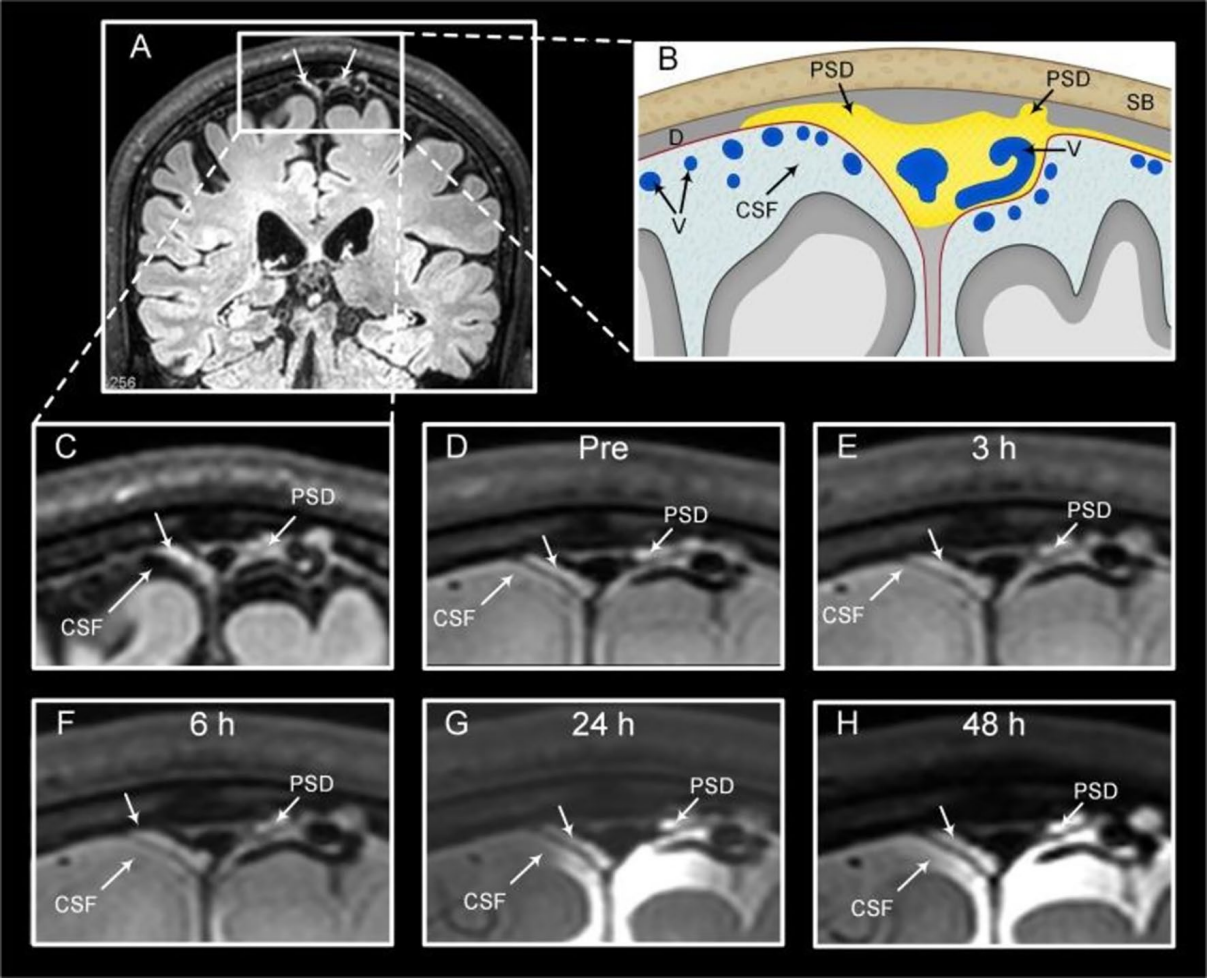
The parasagittal dura (PSD) is visible as hyperintense tissue at FLAIR (A and C). PSD (B) volume varies widely between subjects for unknown reasons, but has in addition to stroma been shown to contain arachnoid granulations, blood vessels, intradural channels, lateral lacunae and lymphatic vessels, the latter at micrometer scale. After injection of a CSF tracer (MRI contrast agent), PSD enhances visibly with tracer after 24 and 48 h in a diffuse fashion, but not in the shape of tubular structures. Tracer enhancement in PSD is dependent on tracer enhancement in the adjacent cerebrospinal fluid (CSF) space and is not visible before tracer has reached the CSF compartment (D–F). At 24 h (G) and 48 h (H),  From Eide PK and Ringstad (Brain Research) [28], reused with permission under the terms of the Creative Commons CC-BY license
